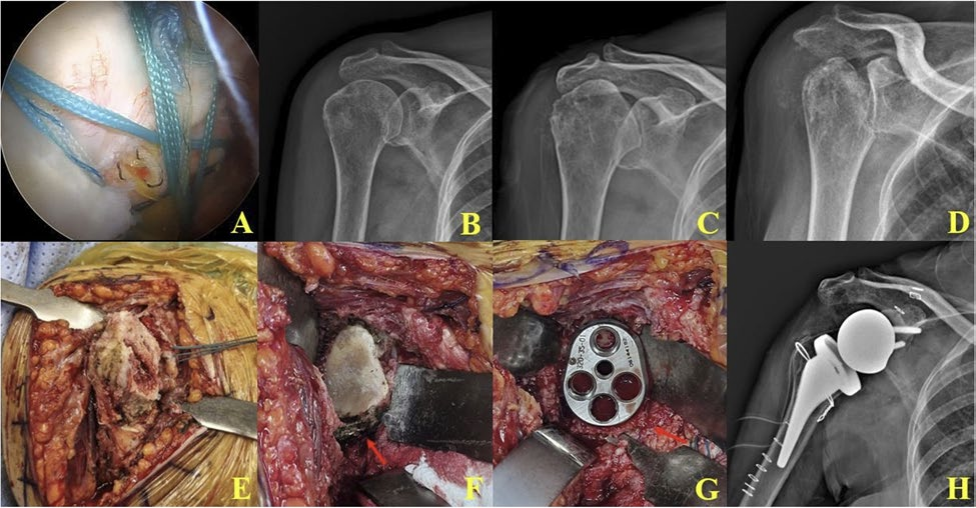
Sequential radiologic, arthroscopic, and intraoperative findings in a patient with rapidly progressive destructive arthropathy (RPDA) undergoing reverse total shoulder arthroplasty (RTSA). A, B Arthroscopic view six months prior shows a suture bridge rotator cuff repair, and plain radiographs demonstrate a normal glenohumeral joint without humeral head collapse. C, D Serial plain radiographs over a four-week period show progressive humeral head collapse and subchondral bone destruction. E Intraoperative photograph reveals extensive subchondral bone loss with severe humeral head destruction. F A secondary glenoid bone defect is observed intraoperatively (red arrow). G Glenoid baseplate fixation is performed with bone grafting (arrow) during the RTSA procedure. H Postoperative radiograph shows a well-positioned reverse total shoulder prosthesis
